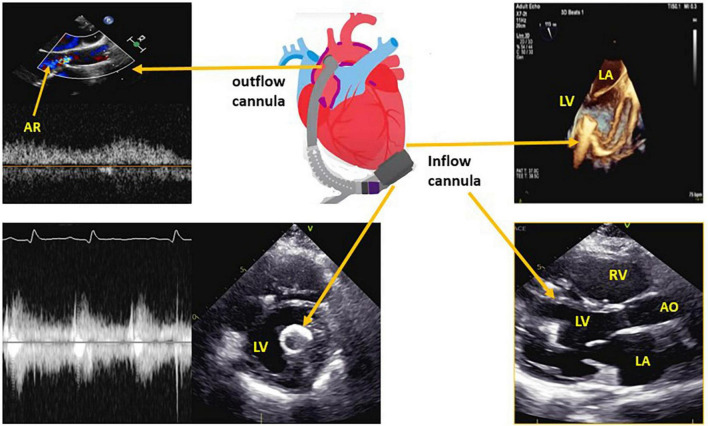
Left ventricular assist device (LVAD) structure with the corresponding echocardiographic images for the inflow cannula and outflow cannula. LV, left ventricle; LA, left atrium; RV, right ventricle; AR, aortic regurgitation.
